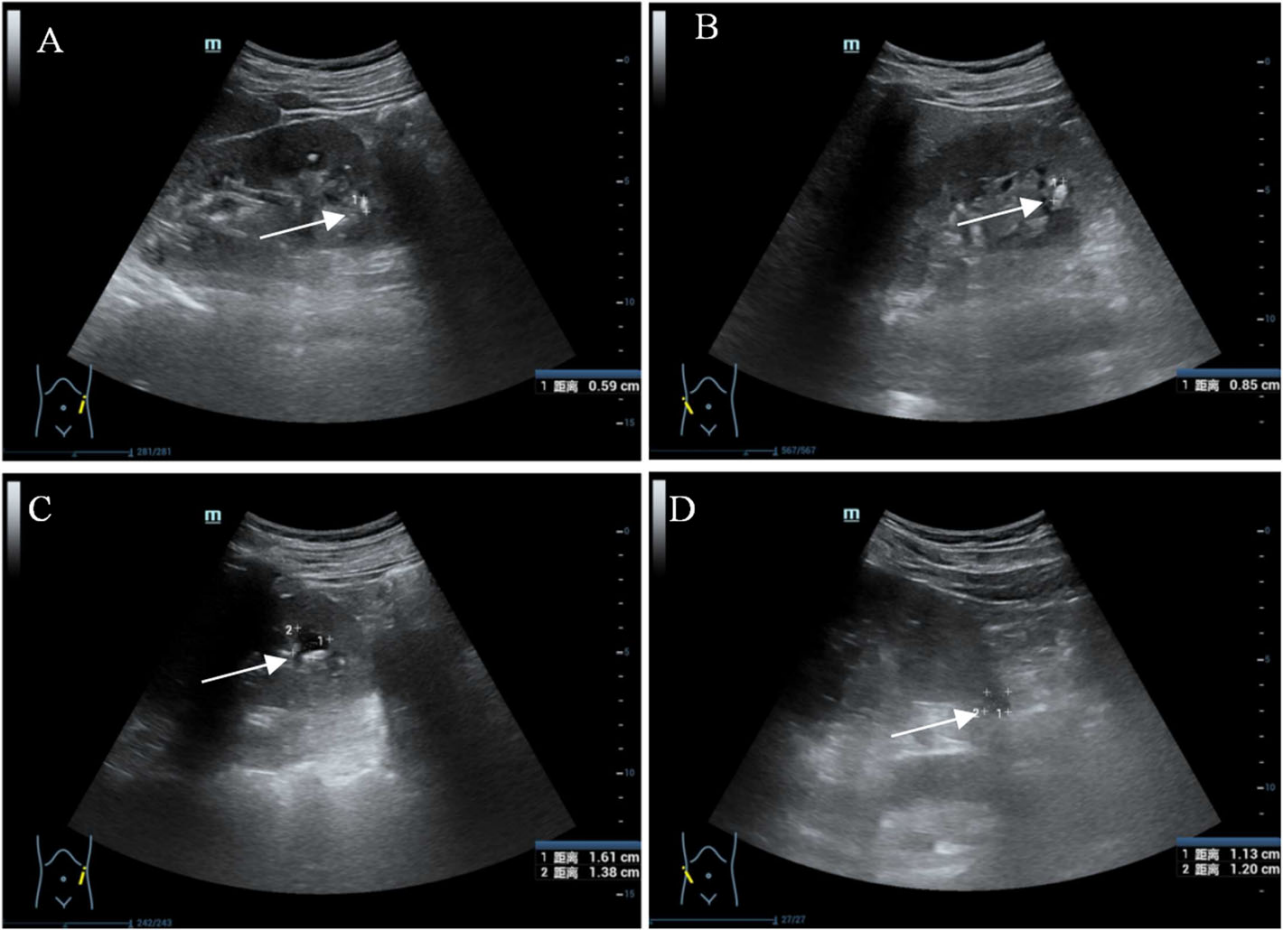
Ultrasound imaging of the urinary tract. a multiple kidney stones in the left kidney (arrow). b multiple kidney stones in the right kidney (arrow). c multiple kidney cysts in the left kidney (arrow). d multiple kidney cysts in the right kidney (arrow)
